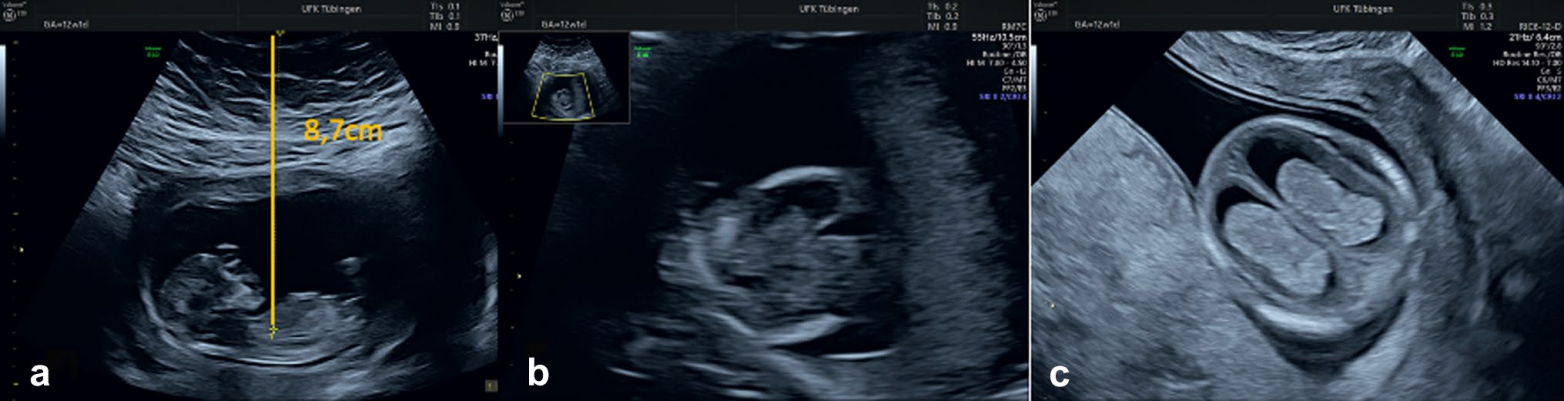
Ultrasound images of a fetus at 12 gestational weeks in a patient with a BMI of 38 kg/m2. Image a shows the crown-rump length and the distance between the fetus and the maternal skin of 8.7 cm. image b and c show the head in the same transverse section, obtained transabdominally (b) and transvaginally (c)
